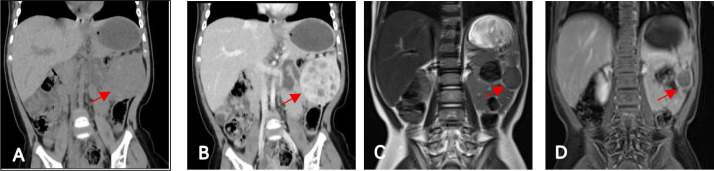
(A) Non-contrast CT scan reveals a round, slightly hypodense mass at the inferior pole of the spleen with well-defined borders, measuring approximately 4.5 cm×4.7 cm×4.6 cm. (B) The spleen mass demonstrates marked peripheral enhancement with multiple internal hypodense areas showing no enhancement. (C, D) At 9 months post-treatment, follow-up MRI revealed a round abnormal signal lesion within the spleen measuring approximately 2.2 cm×2.1 cm×2.2 cm, showing a significant reduction in size compared to previous findings. The lesion had well-defined margins, exhibited slightly hypointense signal intensity on T2WI, and demonstrated marked periphery enhancement on T1-weighted contrast-enhanced scanning.
